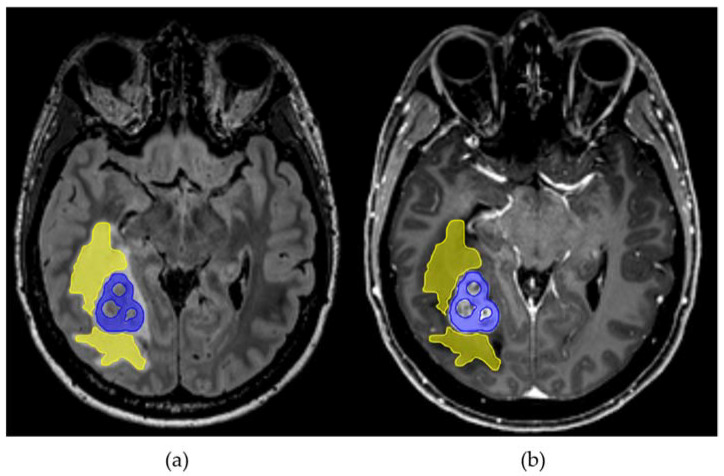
Axial FLAIR (a) and T1 MPRAGE post-Gd (b) images in a patient with a right occipital brain metastasis with corresponding perilesional FLAIR-hyperintensity (yellow) and contrast-enhancing tumor (blue) segmentations.
